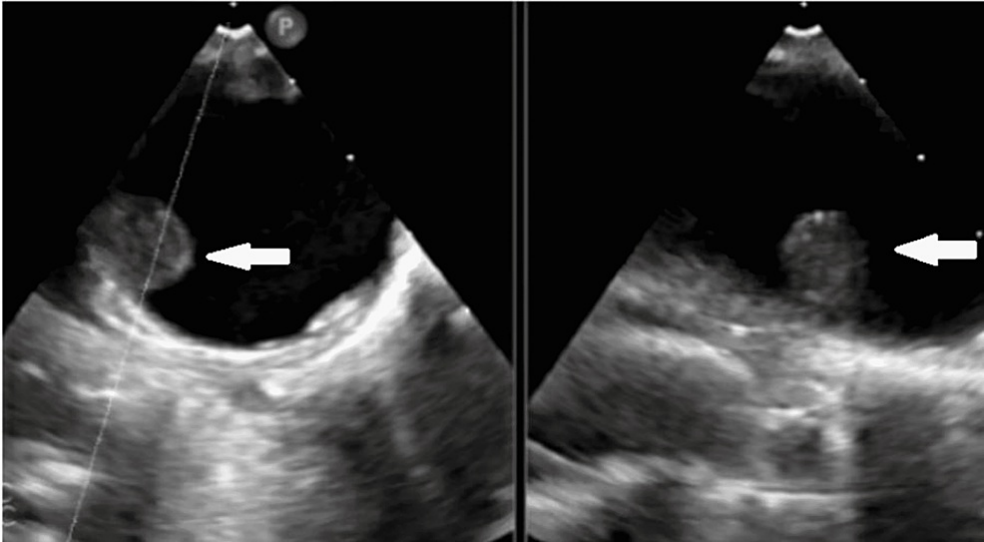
Transesophageal Echocardiogram (TEE) 1x1 cm mobile thrombus at the junction of the distal aortic arch-thoracic aorta.
